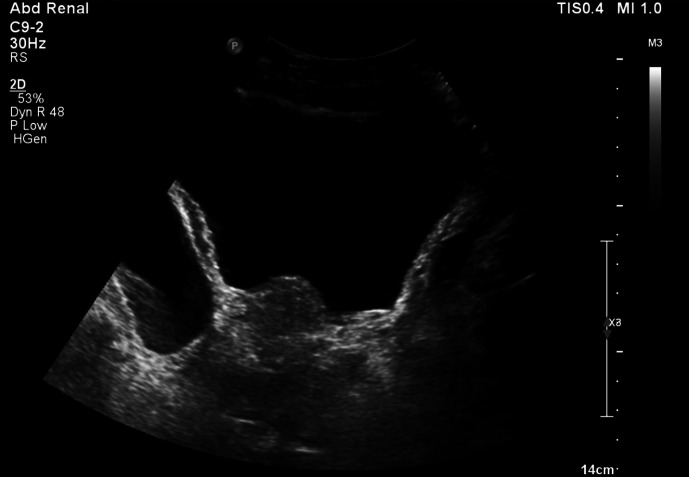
Ultrasound image of the pelvis/lower abdomen (performed prior to admission)
